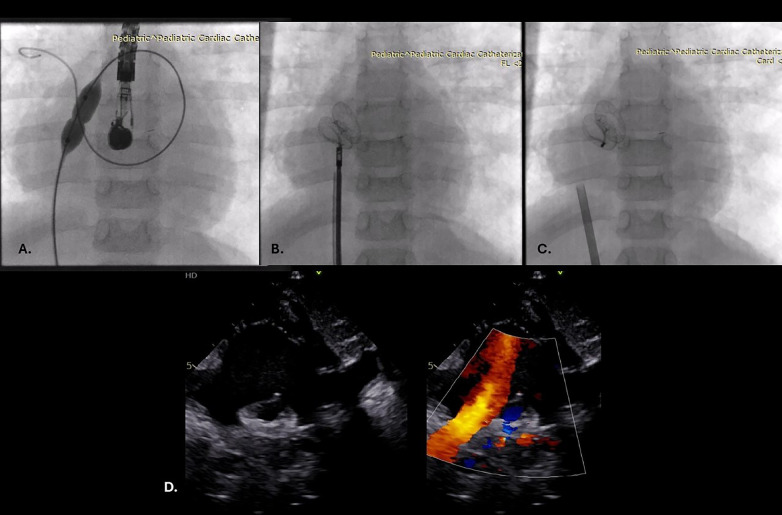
Atrial septal defect predilatation using Z-Med 2 balloon 10 × 20 mm (A); atrial flow regulator (AFR) 8 × 5 mm connected to the delivery cable (B); released AFR (C) echocardiographic examination revealed good device position with bidirectional flow (D).
